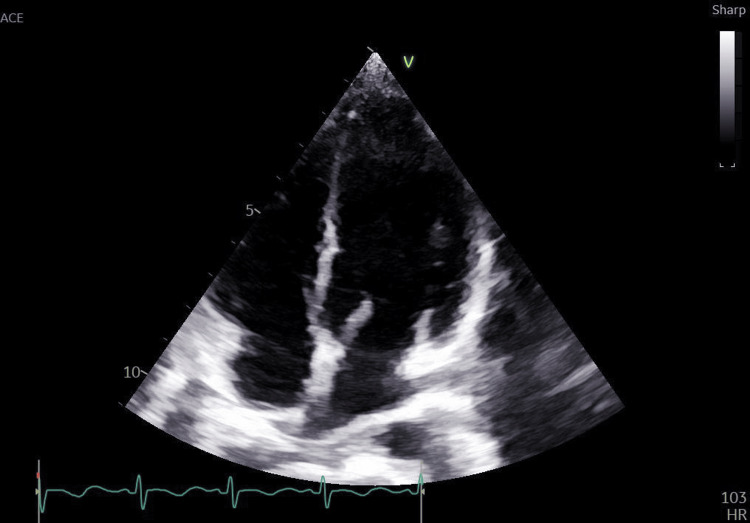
2D echo (apical four-chamber view, suggestive of normal chamber dimensions)
